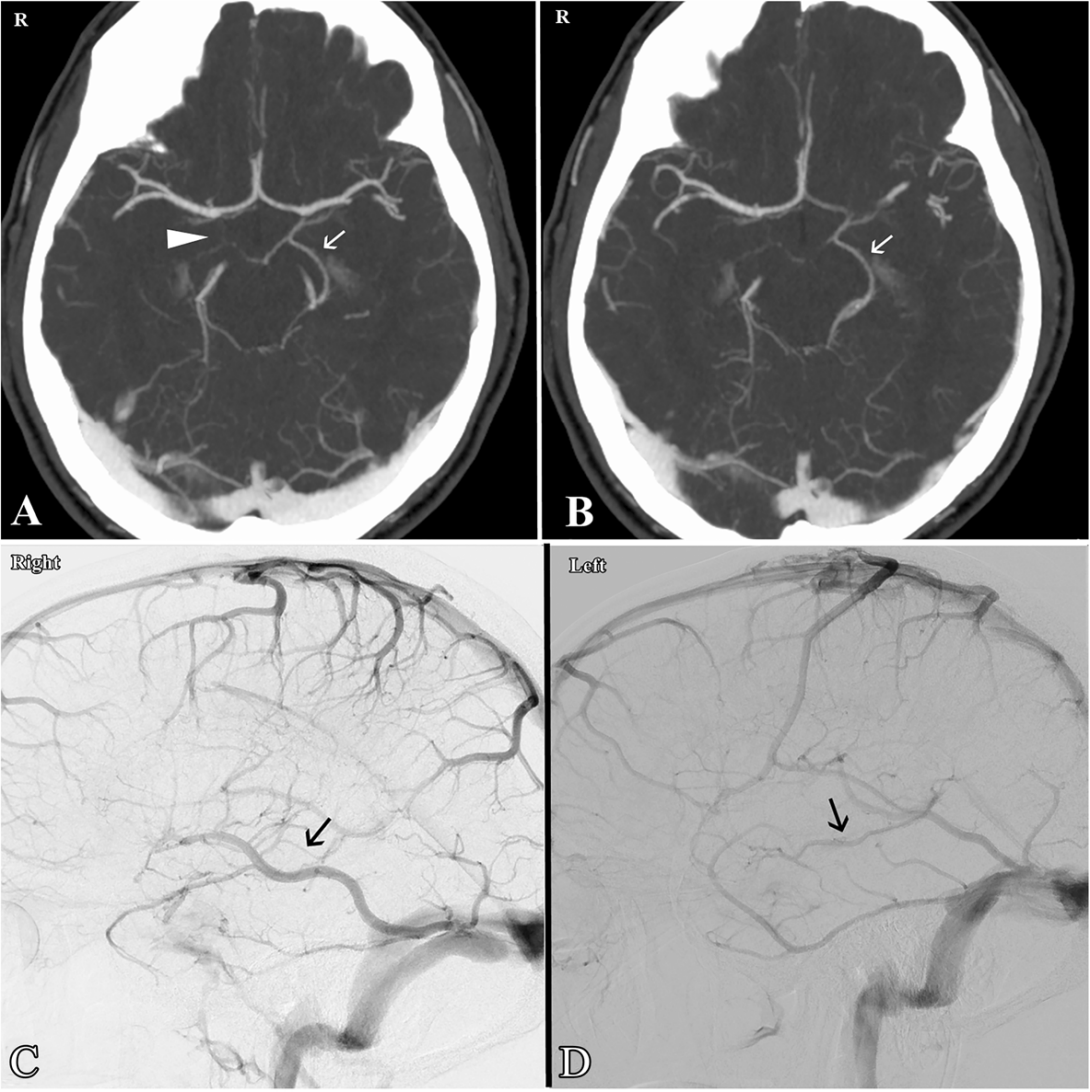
Case 2. Axial computed tomography angiography of the head (A and B) shows a Type B basal vein of Rosenthal on the right side (arrow head in A) and a Type A basal vein of Rosenthal on the left side (arrows in A and B). Lateral view of the venous phase of the internal carotid artery angiogram (C and D) shows a Type B basal vein of Rosenthal on the right side (arrow in C; the basal vein of Rosenthal is not visualized anteriorly) and a Type A basal vein of Rosenthal on the left side (arrow in D).
